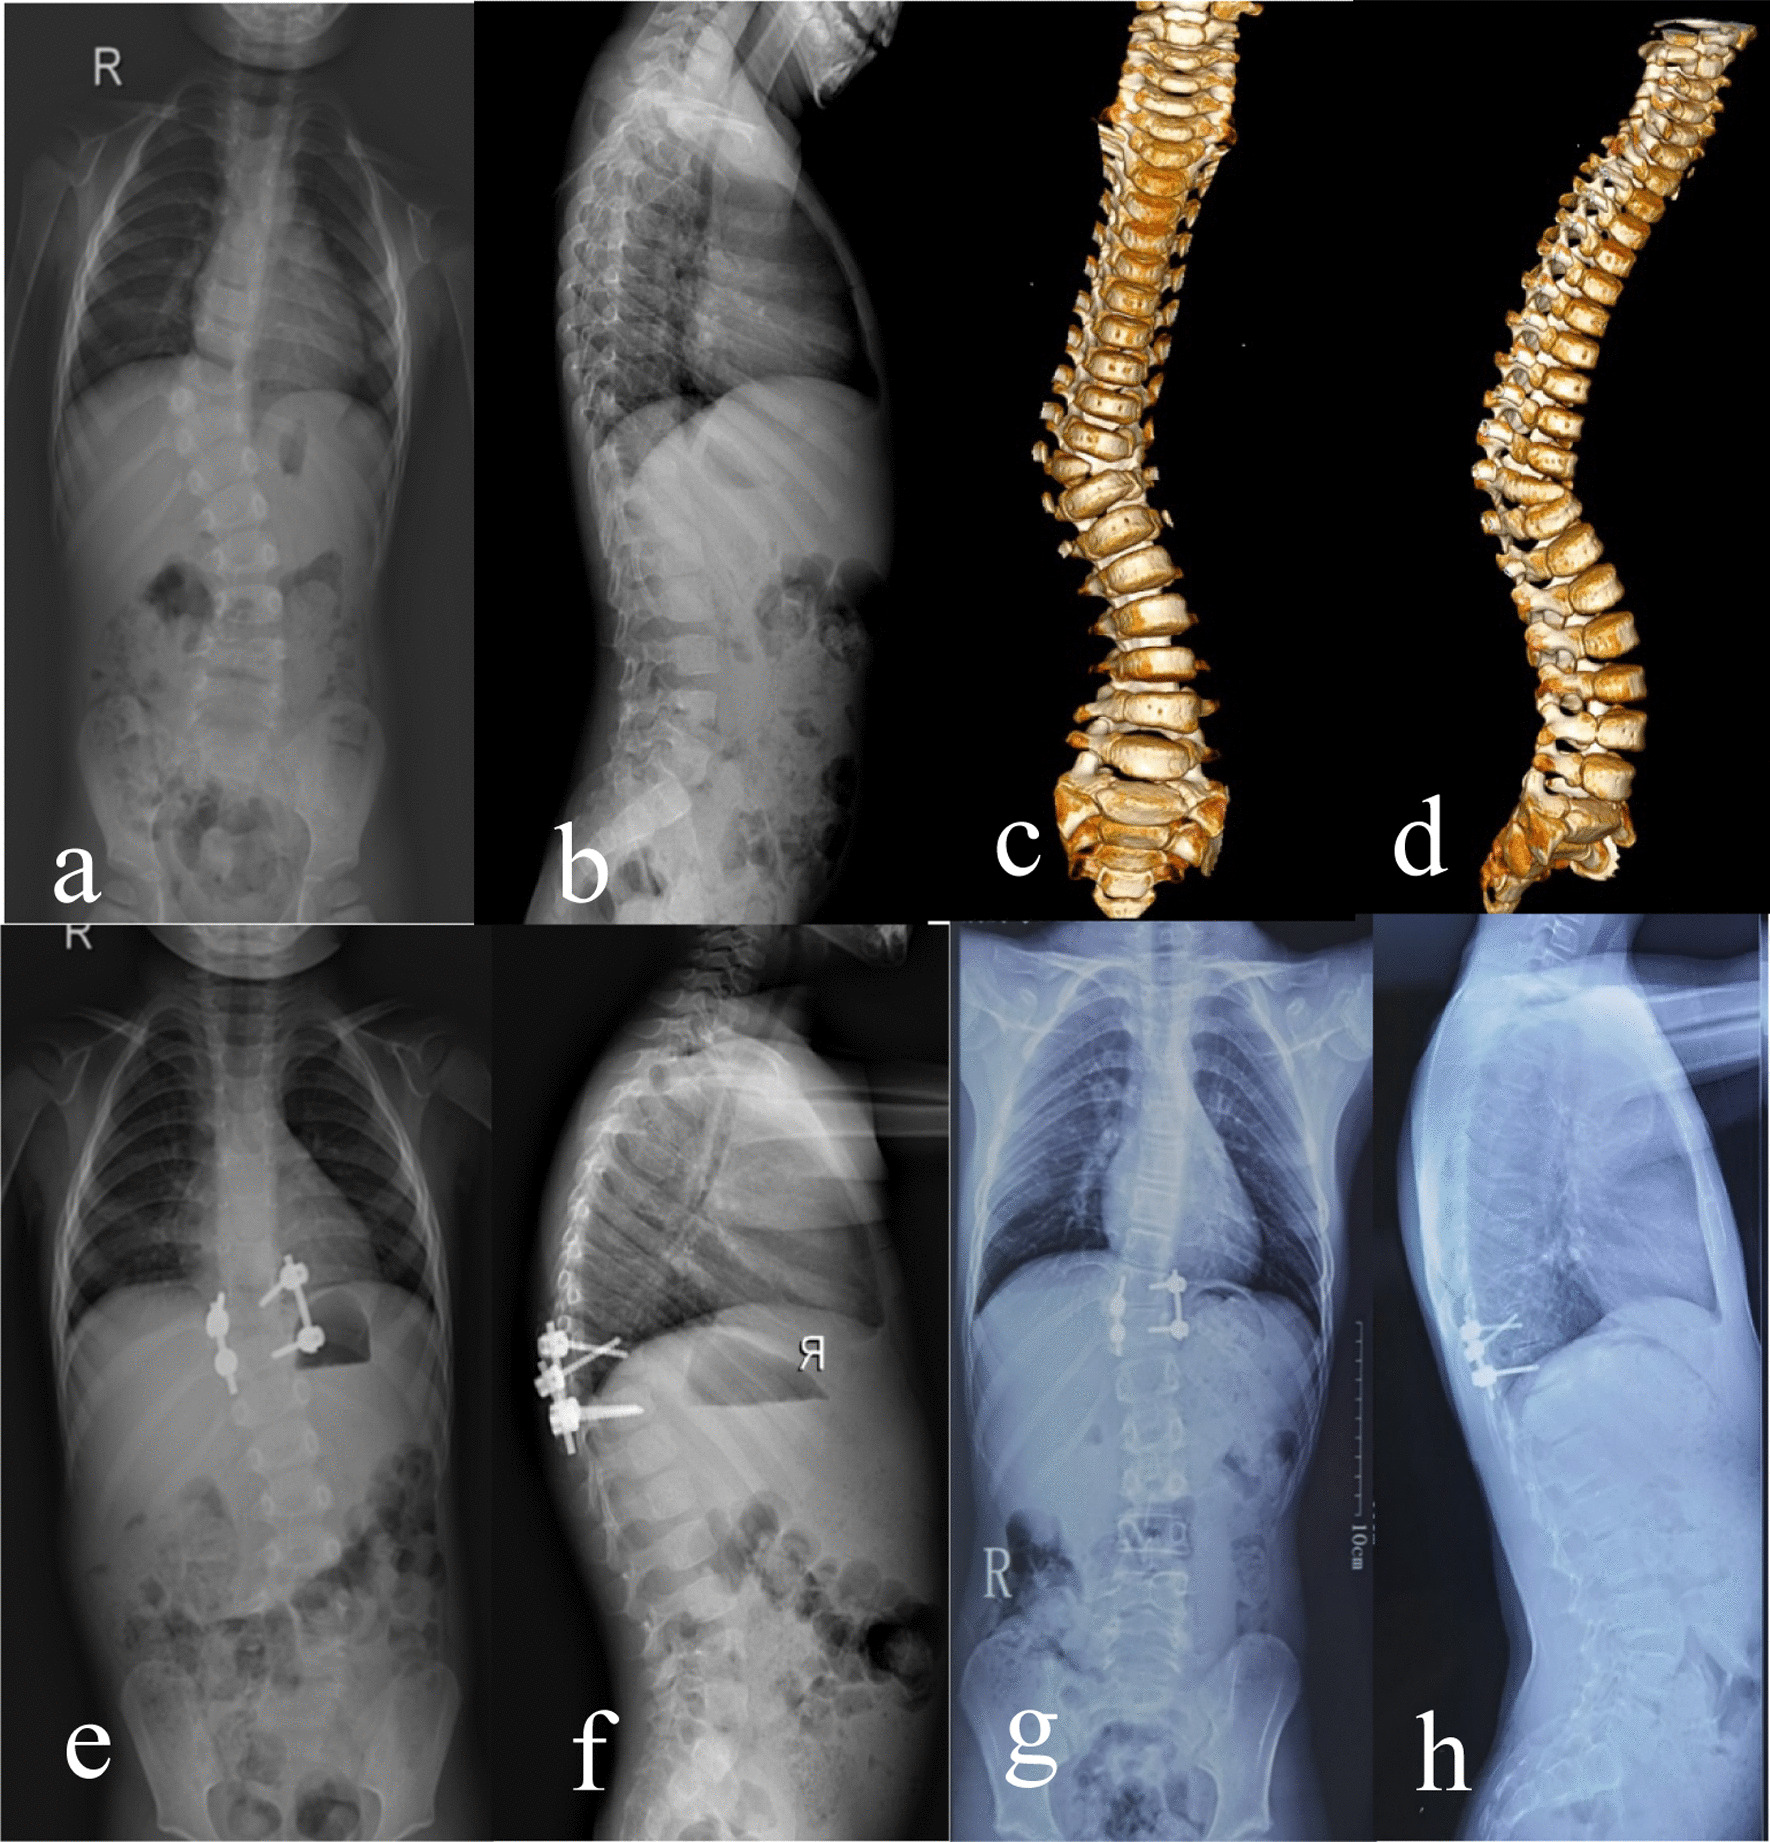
A 3 years 2 months boy with nonincarcerated thoracolumbar hemivertebra. Radiographs obtained preoperatively (a, b, X ray), (c, d, CT scan), postoperatively (e, f) and at the latest follow-up, 8 years later (g, h)
